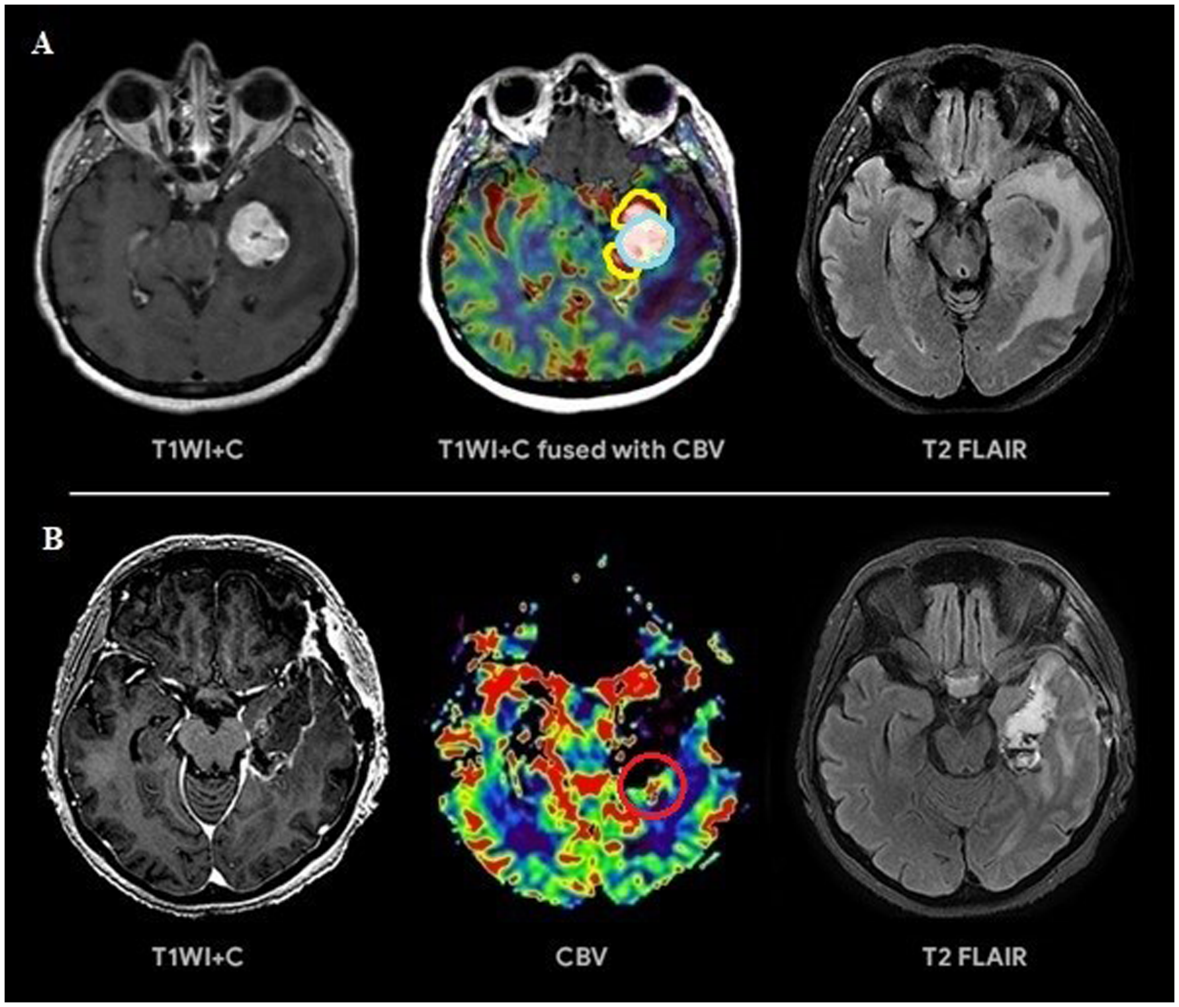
(A) Preoperative tumor resection planning in 52-year-old patient with left temporal lobe glioblastoma with regard to comparison of postcontrast T1 series, CBV parameter obtained using DSC-T2* perfusion and T2-Flair. The tumor compounds of 2 portions: contrast-accumulating (blue line) and contrast-negative with high CBV level (yellow line). (B) Postoperative MRI control 24 h after operation, post-contrast T1 series, CBV indicator obtained using DSC-T2* perfusion and T2-Flair. MRI data indicating resection of the contrast-enhancing tumor part and with residual hyperperfused fragment at the lower pole of resection zone (red circle).
